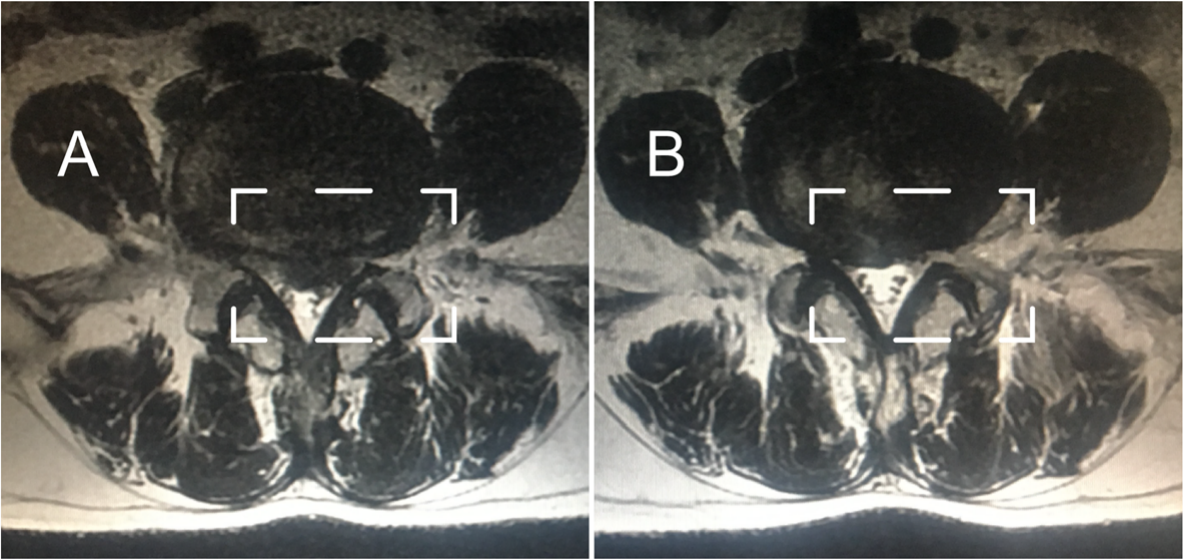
The MRI of patients subjected to PTED with different grades of facetectomy. a Preoperative imagine data. b Postoperative imagine data
